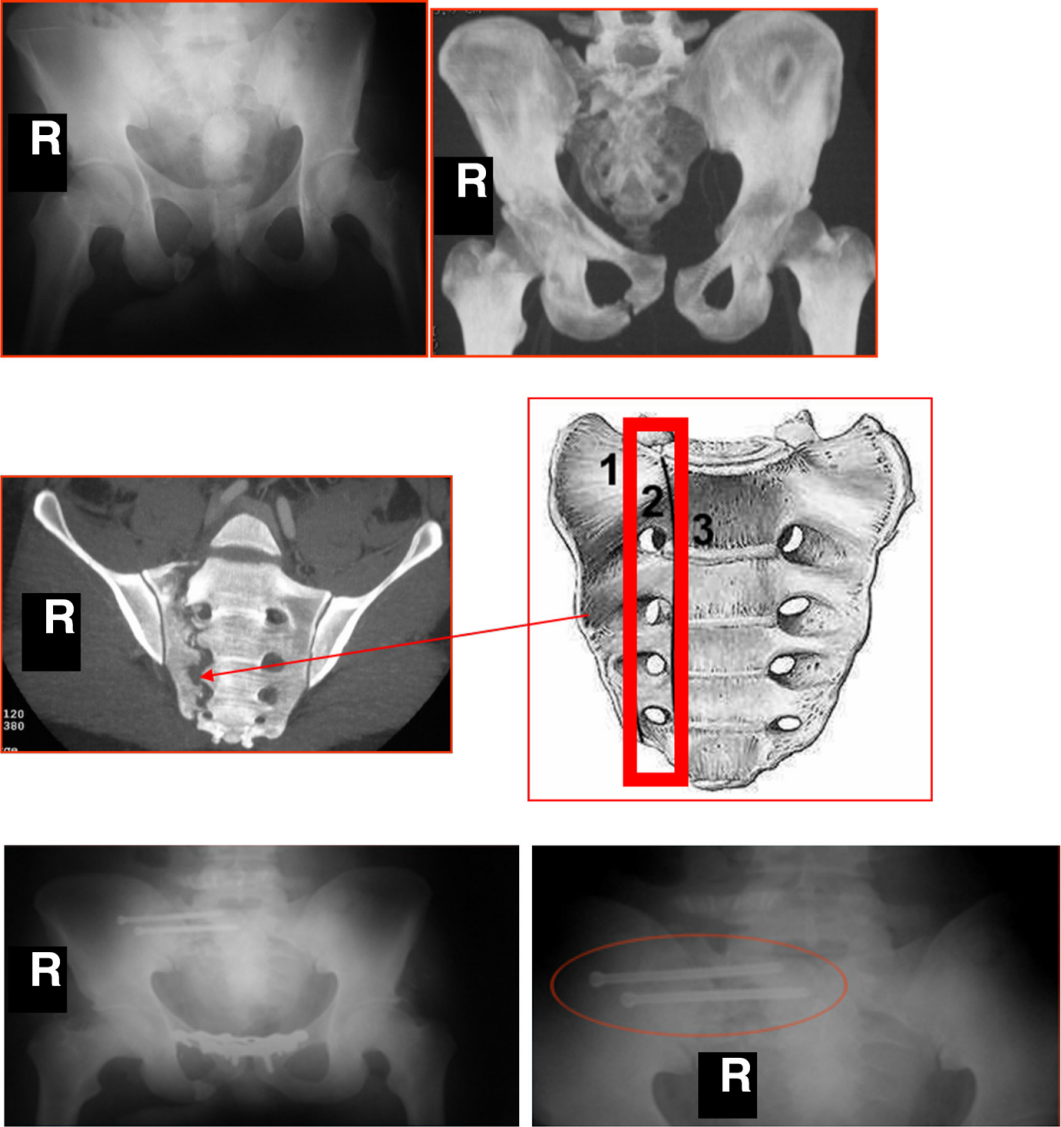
Case of a 19-year-old male patient who sustained a Type 2 Dennis fracture of the sacrum associated with pubic symphysis diastasis and superior subluxation of the right side of the pelvis (type C1) following a fall from height (top pictures: AP radiographic view and pelvis CT-reconstruction; middle pictures: coronal view). The patient was initially treated with reduction and fixation with double plate and screws (anterior stabilization with anterior approach). This was followed by S-I joint fixation with two percutaneous CT-guided screws within S1 and S2 vertebral body 5 days following the initial procedure (bottom pictures: plain radiographs following percutaneous screw fixation).
